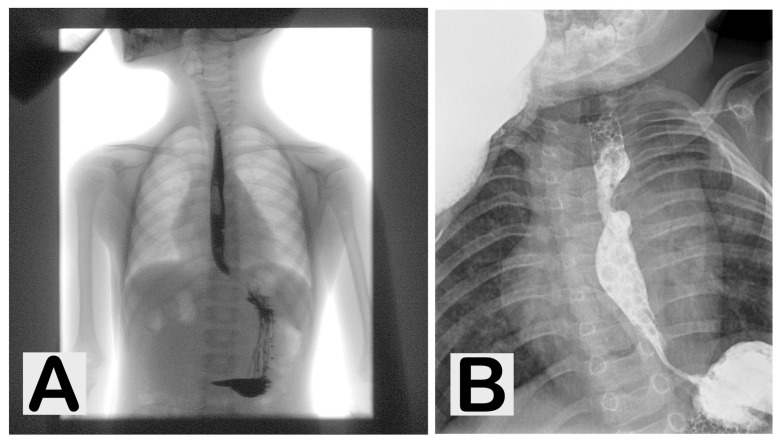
Contrast-enhanced esophagograms in pediatric patients. (A) Normal esophageal transit with continuous contrast column and no evidence of stricture or dilation. (B) Radiographic image showing esophageal stricture with proximal dilation and impaired passage of contrast medium, suggestive of an anastomotic complication.
